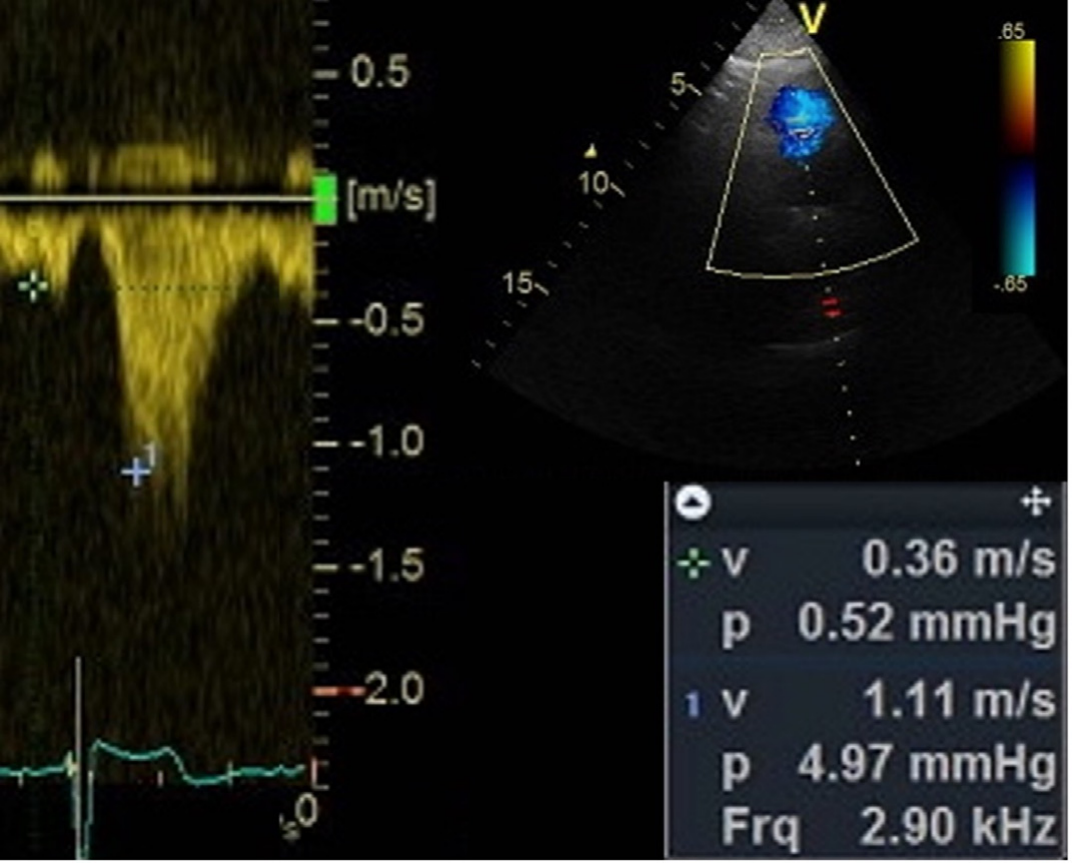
Left Ventricular Assist Device The systolic-diastolic velocity measurements are sampled in the outflow canula by pulsed-wave Doppler imaging from the right parasternal view.
